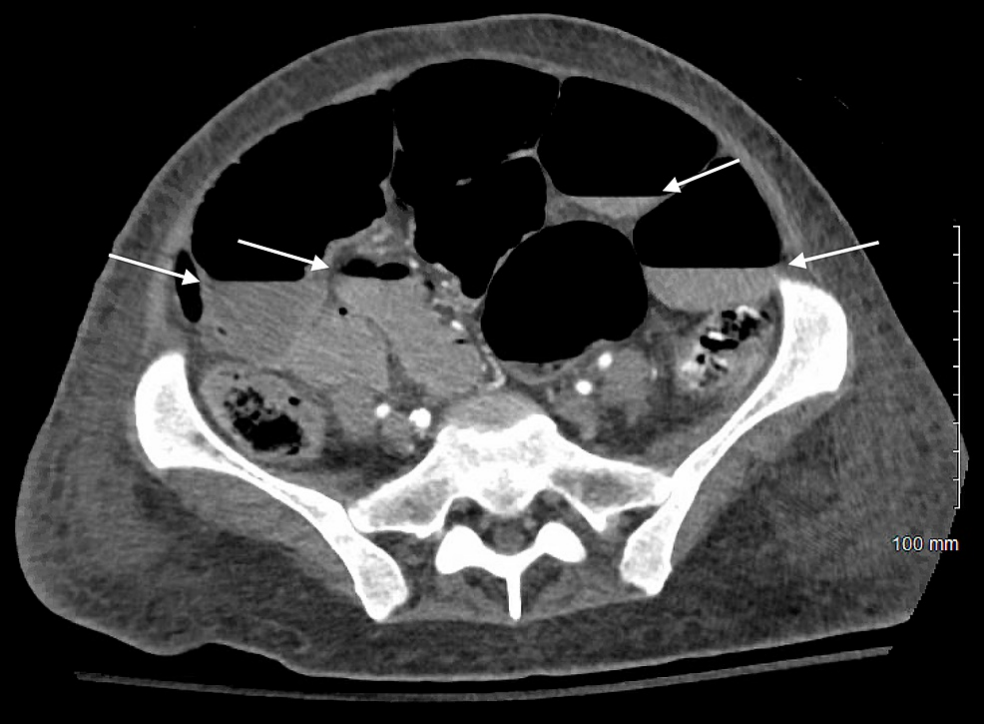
CT image of air-fluid levels from the patient's second high-grade distal small bowel obstruction (arrows) CT: computed tomography
